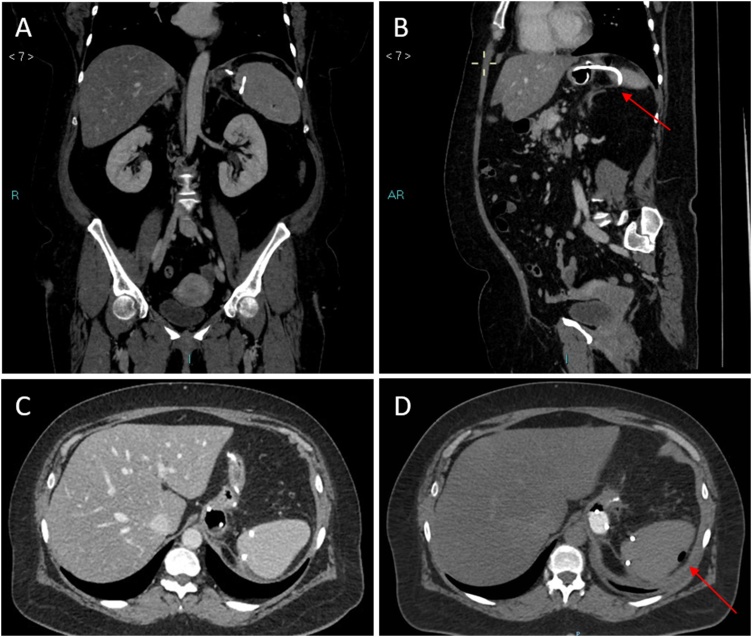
(A– D) – Abdominal computed tomographic scan with intravenous contrast, showing partial intrasplenic displacement of the double-pigtail stent, more evident in oblique projection along the axis of the vessel (red arrow in B). The presence of air in the subcapsular region confirmed intrasplenic displacement of the drain (red arrow in D).
